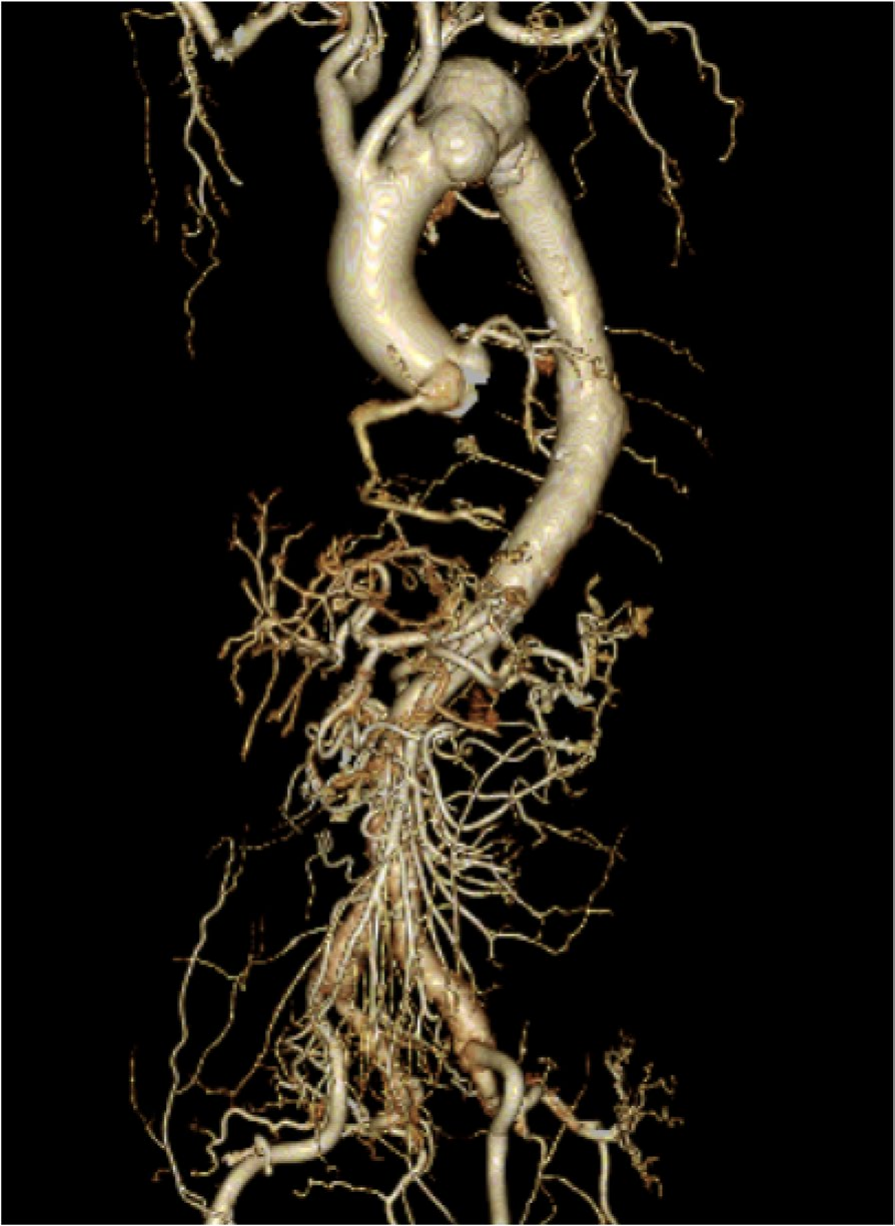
Preoperative 3-dimensional computed tomography (CT) of the aorta and coronary arteries
